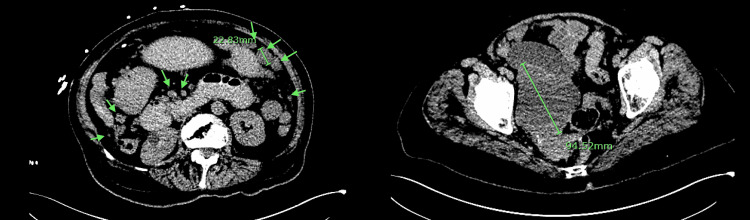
Computed tomography of abdomen pelvis without IV contrast at the time of diagnoses showing multiple masses throughout the abdomen and pelvis, consistent with peritoneal studding and possible primary mass in the right pelvic region which measures 9.4 cm x 7.1 cm
